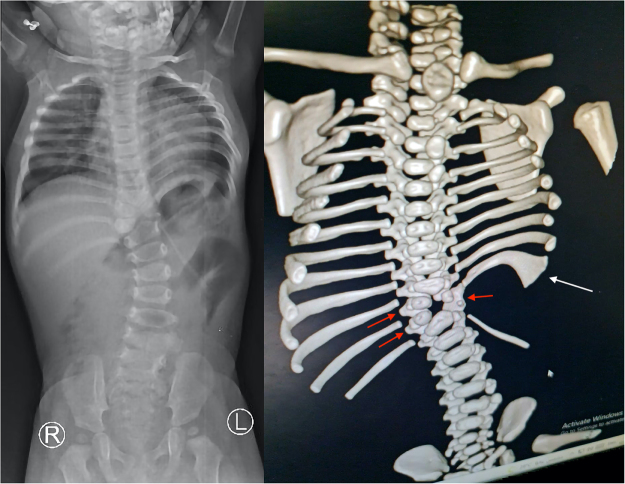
3D CT mainly showing skeletal anomalies, including hemivertebrae at T10 and T11 (red arrows), fused laminae at these levels, congenital fusion of the left 10th and 11th ribs (white arrow), and scoliosis convex toward the side of the lumbar hernia.
